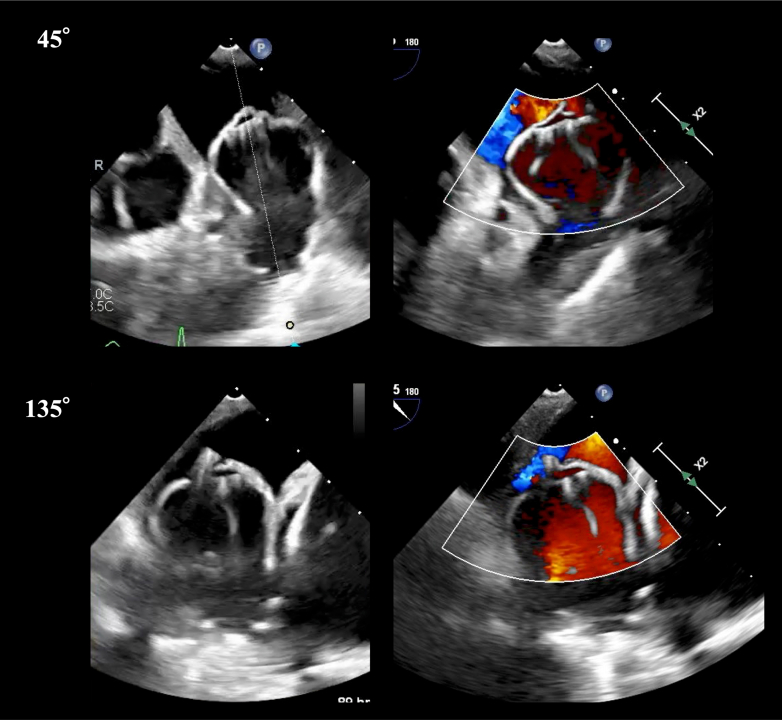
Intraprocedural TEE Findings During LAAO A 40-mm device was deployed according to the preablation plan with intentional overcompression (29%), yielding stable seating and no peridevice leak on color Doppler. LAAO = left atrial appendage occlusion; TEE = transesophageal echocardiography.
